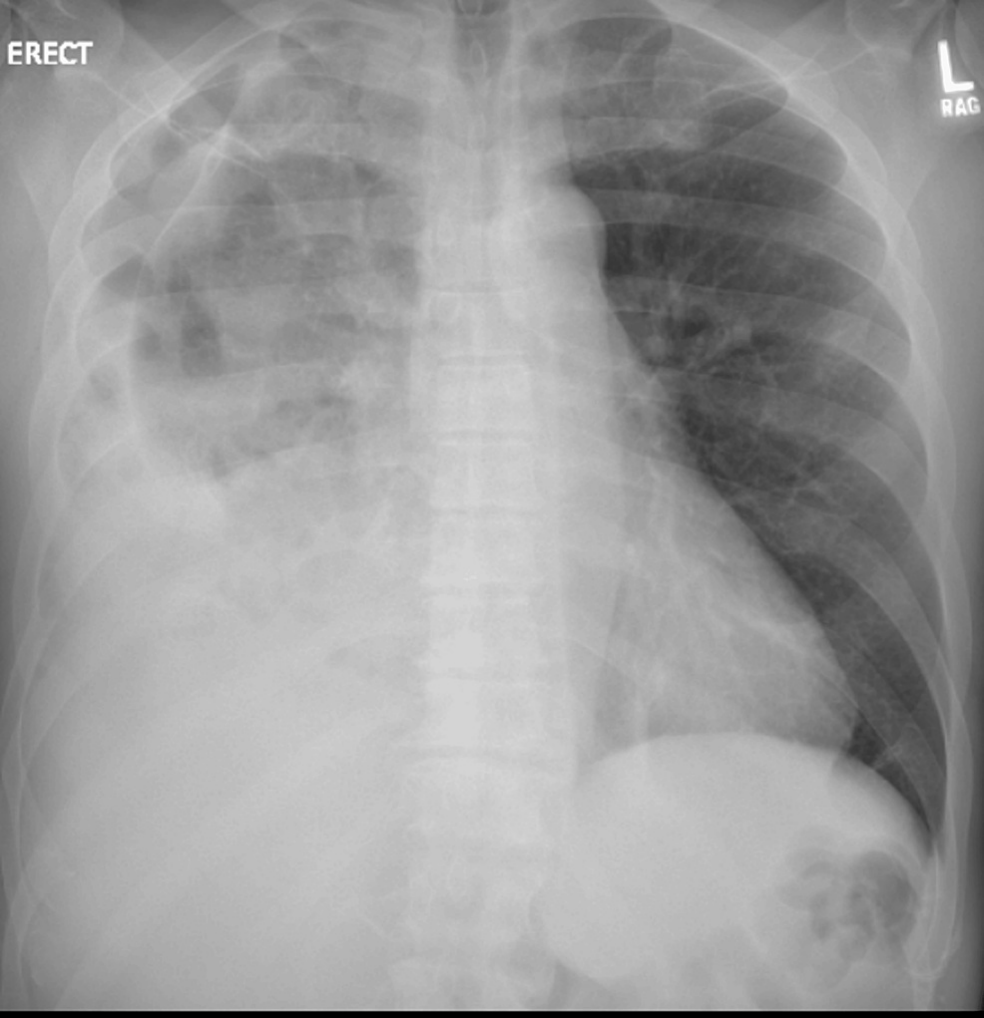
AP chest X-ray showing right moderate-size pneumothorax and a loculated pleural effusion. AP: Anteroposterior
